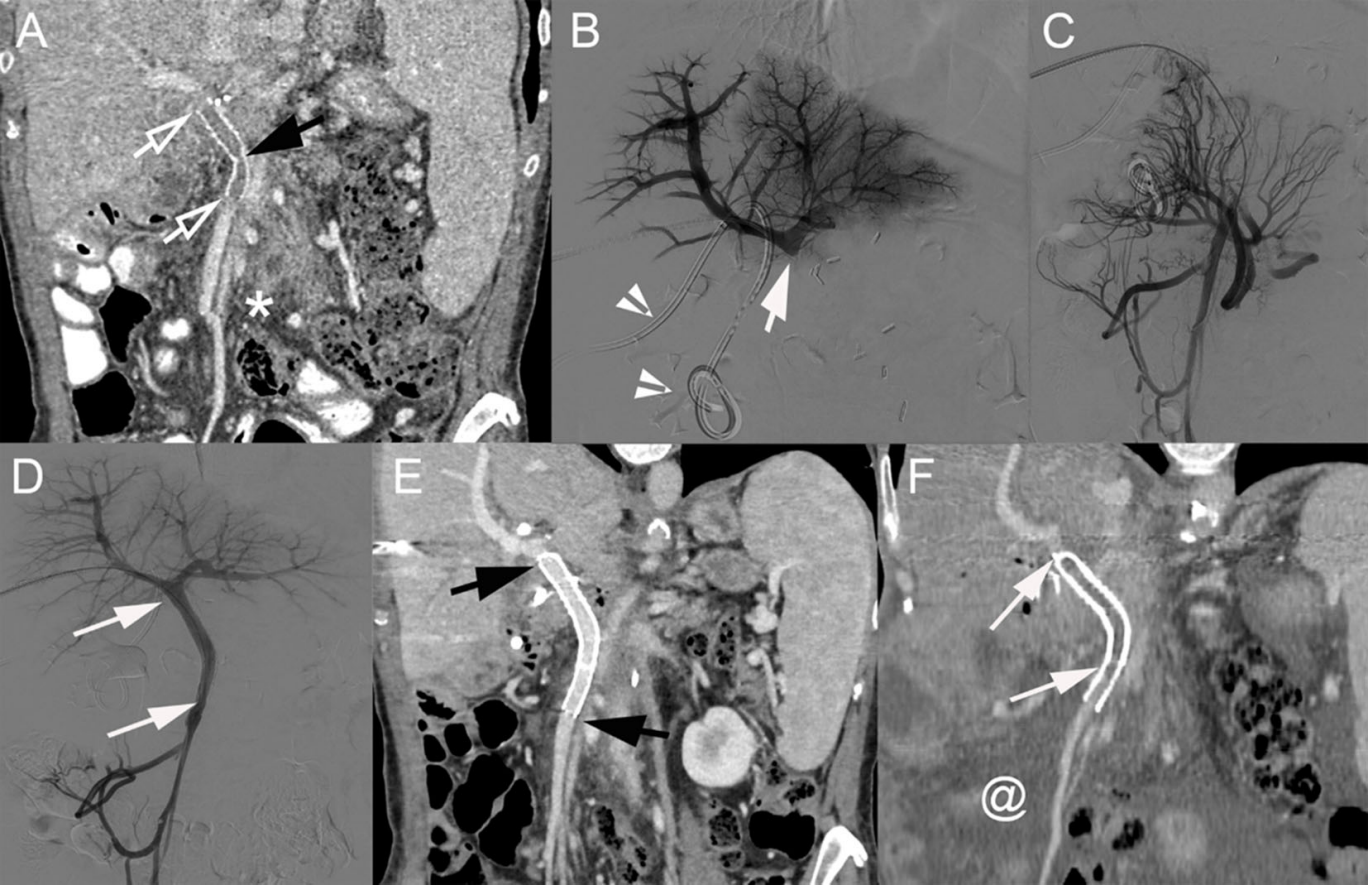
(A) Coronal CT image (portal venous phase) of a 60-year-old woman with pancreatic cancer 6 months after PD with venous resection and prosthetic graft placement between the SMV and PV (below the PV bifurcation). The prosthetic graft contains hypoattenuating material consistent with thrombosis (hollow arrows). A kink in the graft is present (black arrow). (B) Direct portography showing complete graft occlusion below the PV bifurcation (arrow). Biliary drainage catheter (arrowheads). (C) Venography of the caudal SMV (after crossing the thrombosed graft) showing the development of multiple venous collaterals. (D) After deployment of 2 stents [8 × 40 mm (SMART Flex, Cordis) and 10 × 60 mm (SMART Control, Cordis); arrows], completion mesenteric portography demonstrates complete recanalization and disappearance of venous collaterals. Coronal CT image (portal venous phase) at 1 week (E) and 17 months (F) after percutaneous transhepatic venous graft recanalization and stenting. (E) Stent patency is visible (black arrows). (F) Complete stent thrombosis is demonstrated (arrows) together with the development of marked ascites (@). PD, pancreatoduodenectomy; SMV, superior mesenteric vein; PV, portal vein.
